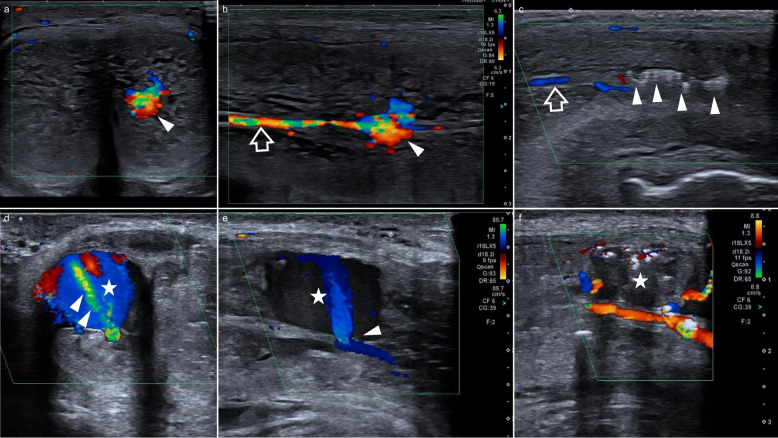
Color-coded Doppler ultrasound of a small arterio-cavernous fistula on the left cavernous body (arrowhead, a + b) with successful coil embolization (c, arrowhead) and denotable change of arterial inflow after treatment (arrow, b + c). In contrast, large right-sided arterio-cavernous fistula (asterisk, d–f) with fanfare-like inflow (arrowhead, d + e) and successful embolization with gelatin (f). Image orientation: a, d: transversal plane; b, c, e, f: longitudinal plane
